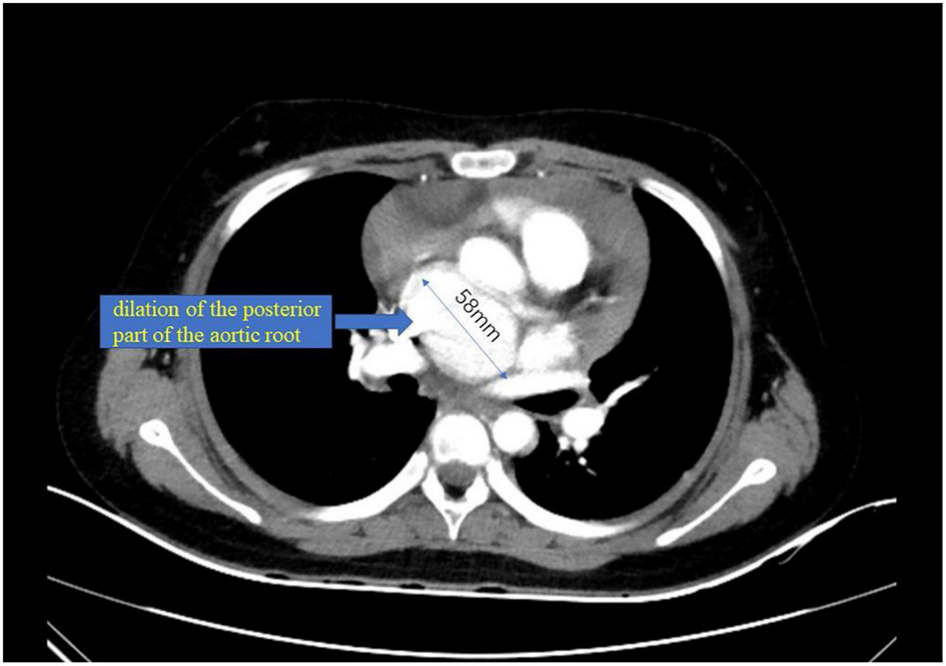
Computed tomography (CT).
